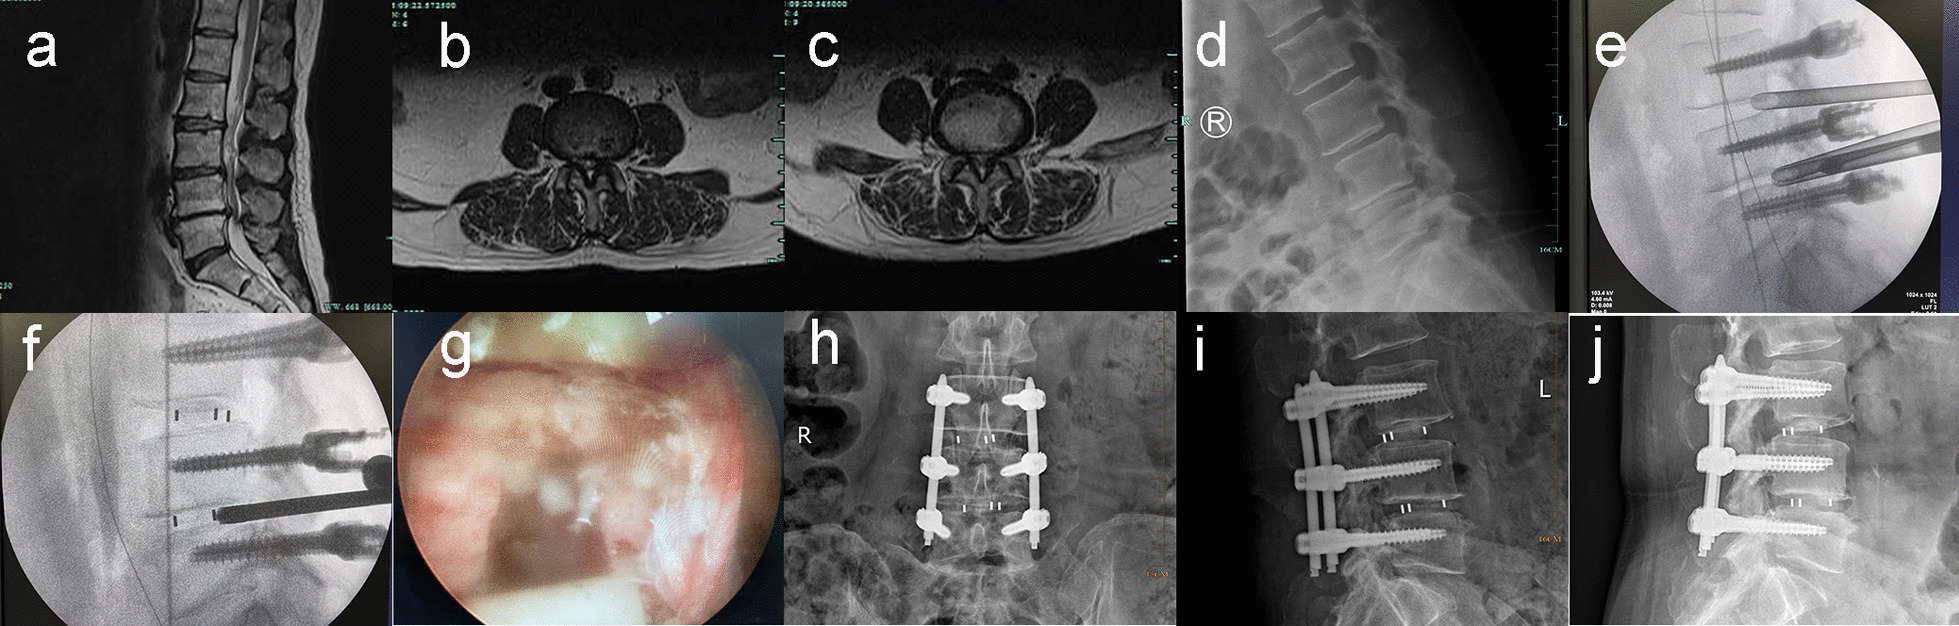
Typical case 3. A 66-year-old female with low back pain for 10 years, aggravating with lower limbs numbness and soreness for six months. Sagittal MRI shows disc herniation at L3/4 and L4/5 levels, and endplate degeneration with reduced intervertebral height at L4/5 level (a). Axial MRI show spinal stenosis at L3/4 and L4/5 levels (b, c). Hyperextension X-ray demonstrates instability at L3/4 and L4/5 levels (d). Place the working channel and perform the foraminoplasty (e). Implant the cage and then make secondary decompression (f, g). Anteroposterior and lateral X-ray one month after surgery (h, i). Lateral X-ray 1 year after surgery (j)
